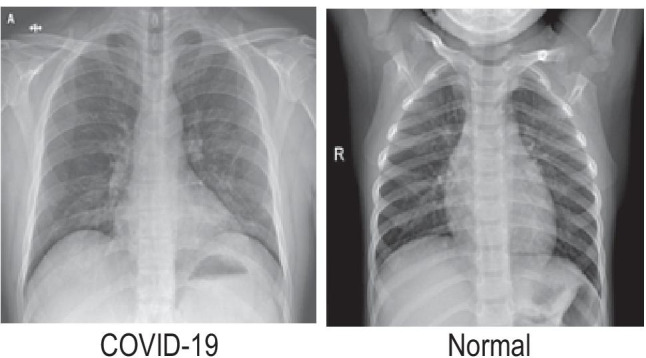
Example chest X-ray images from the dateset – COVID-19 (left), and normal (right)
